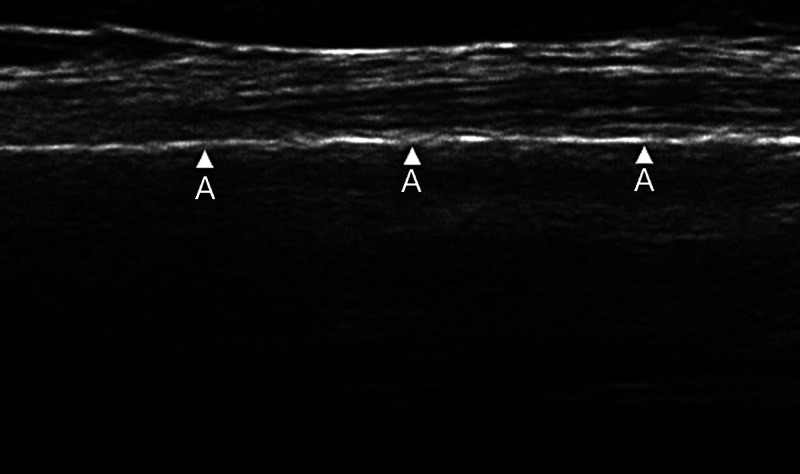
Ultrasound of intact tibial diaphyseal cortex as demonstrated by the arrowheads Ultrasound image from Qadi et al. 2020 [9] A- Intact continuous bony cortex Original images authorised by respective owners
